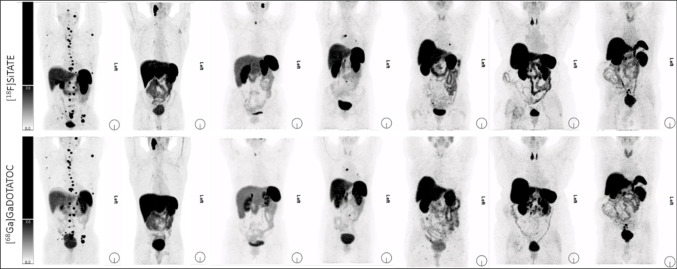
Comparison of [^18^F]SiTATE-PET/CT and previous [^68^Ga]Ga-DOTATOC-PET/CT in 7 patients with near-stable disease and no treatment between the two PET scans. Maximum intensity projections of [^18^F]SiTATE-PET (upper row) and [^68^Ga]Ga-DOTATOC-PET (lower row)
